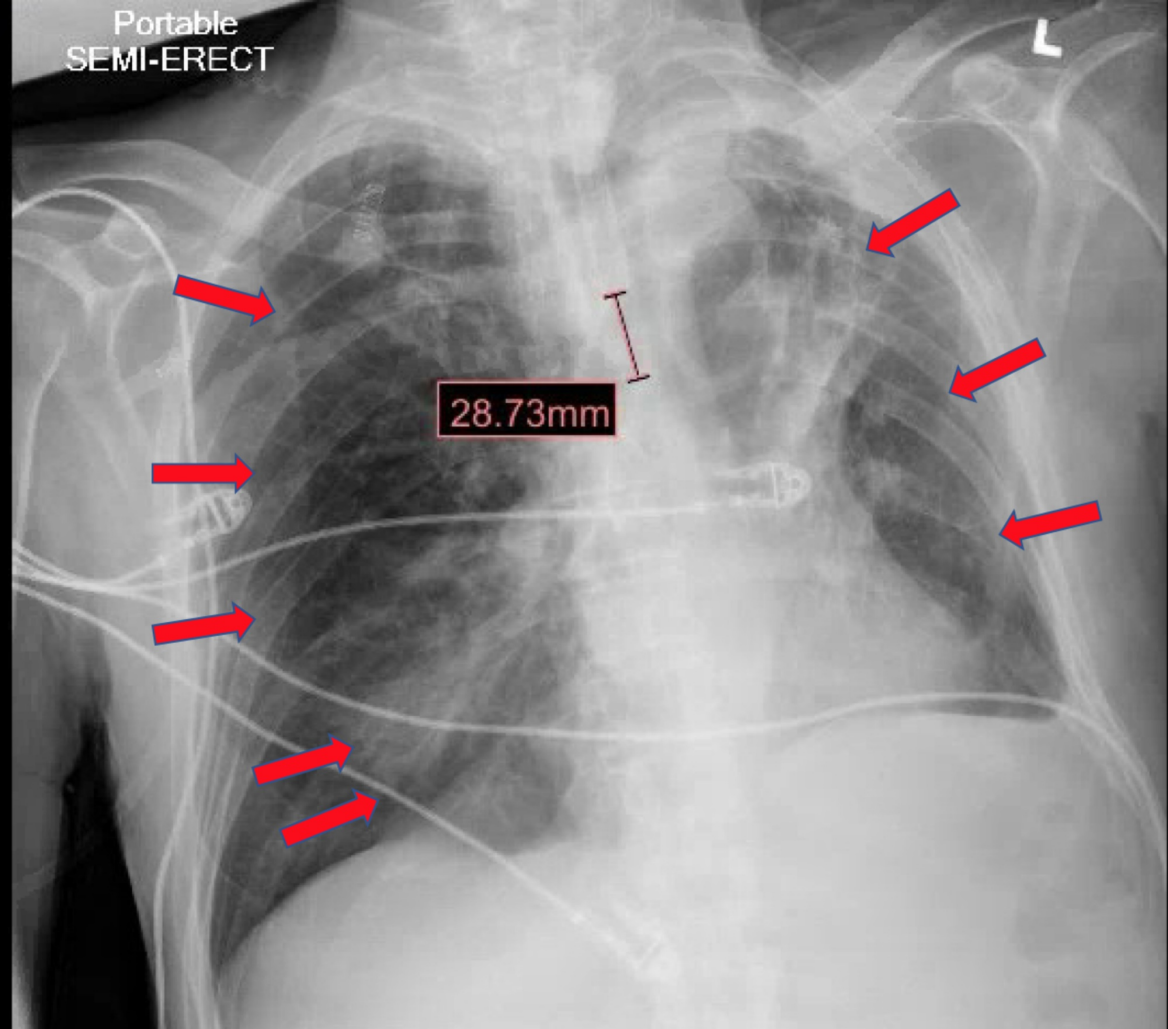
Chest x-ray on Day 5 of hospital course, status-post hydroxychloroquine and azithromycin treatment and just prior to extubation and downgrade from the intensive care unit (ICU), showing improvement of bilateral patchy opacities (arrows) and decreased right lower lobe consolidation (arrows)
